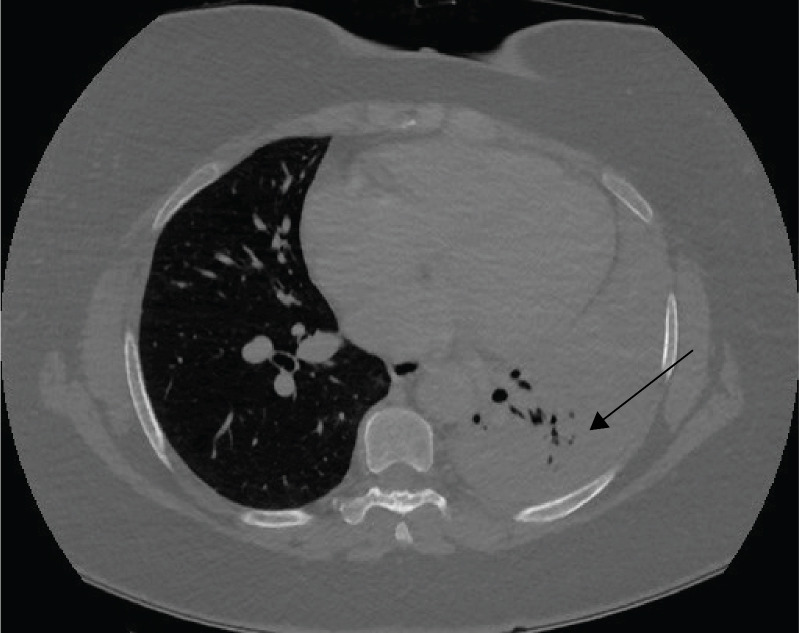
Chest computed tomography image confirming consolidation of the left lung
